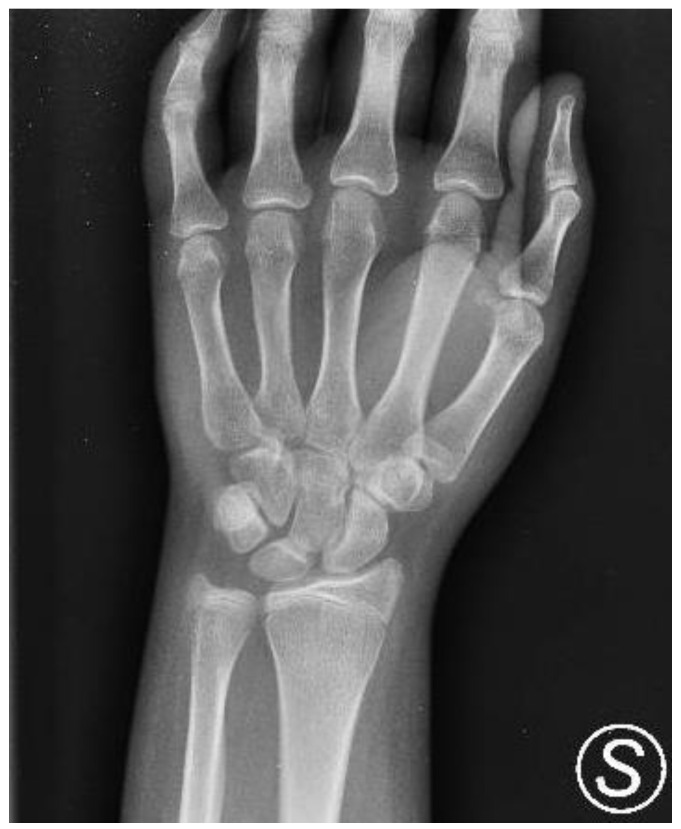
X-ray of the left wrist. Images not attributable to periosteal reaction.
